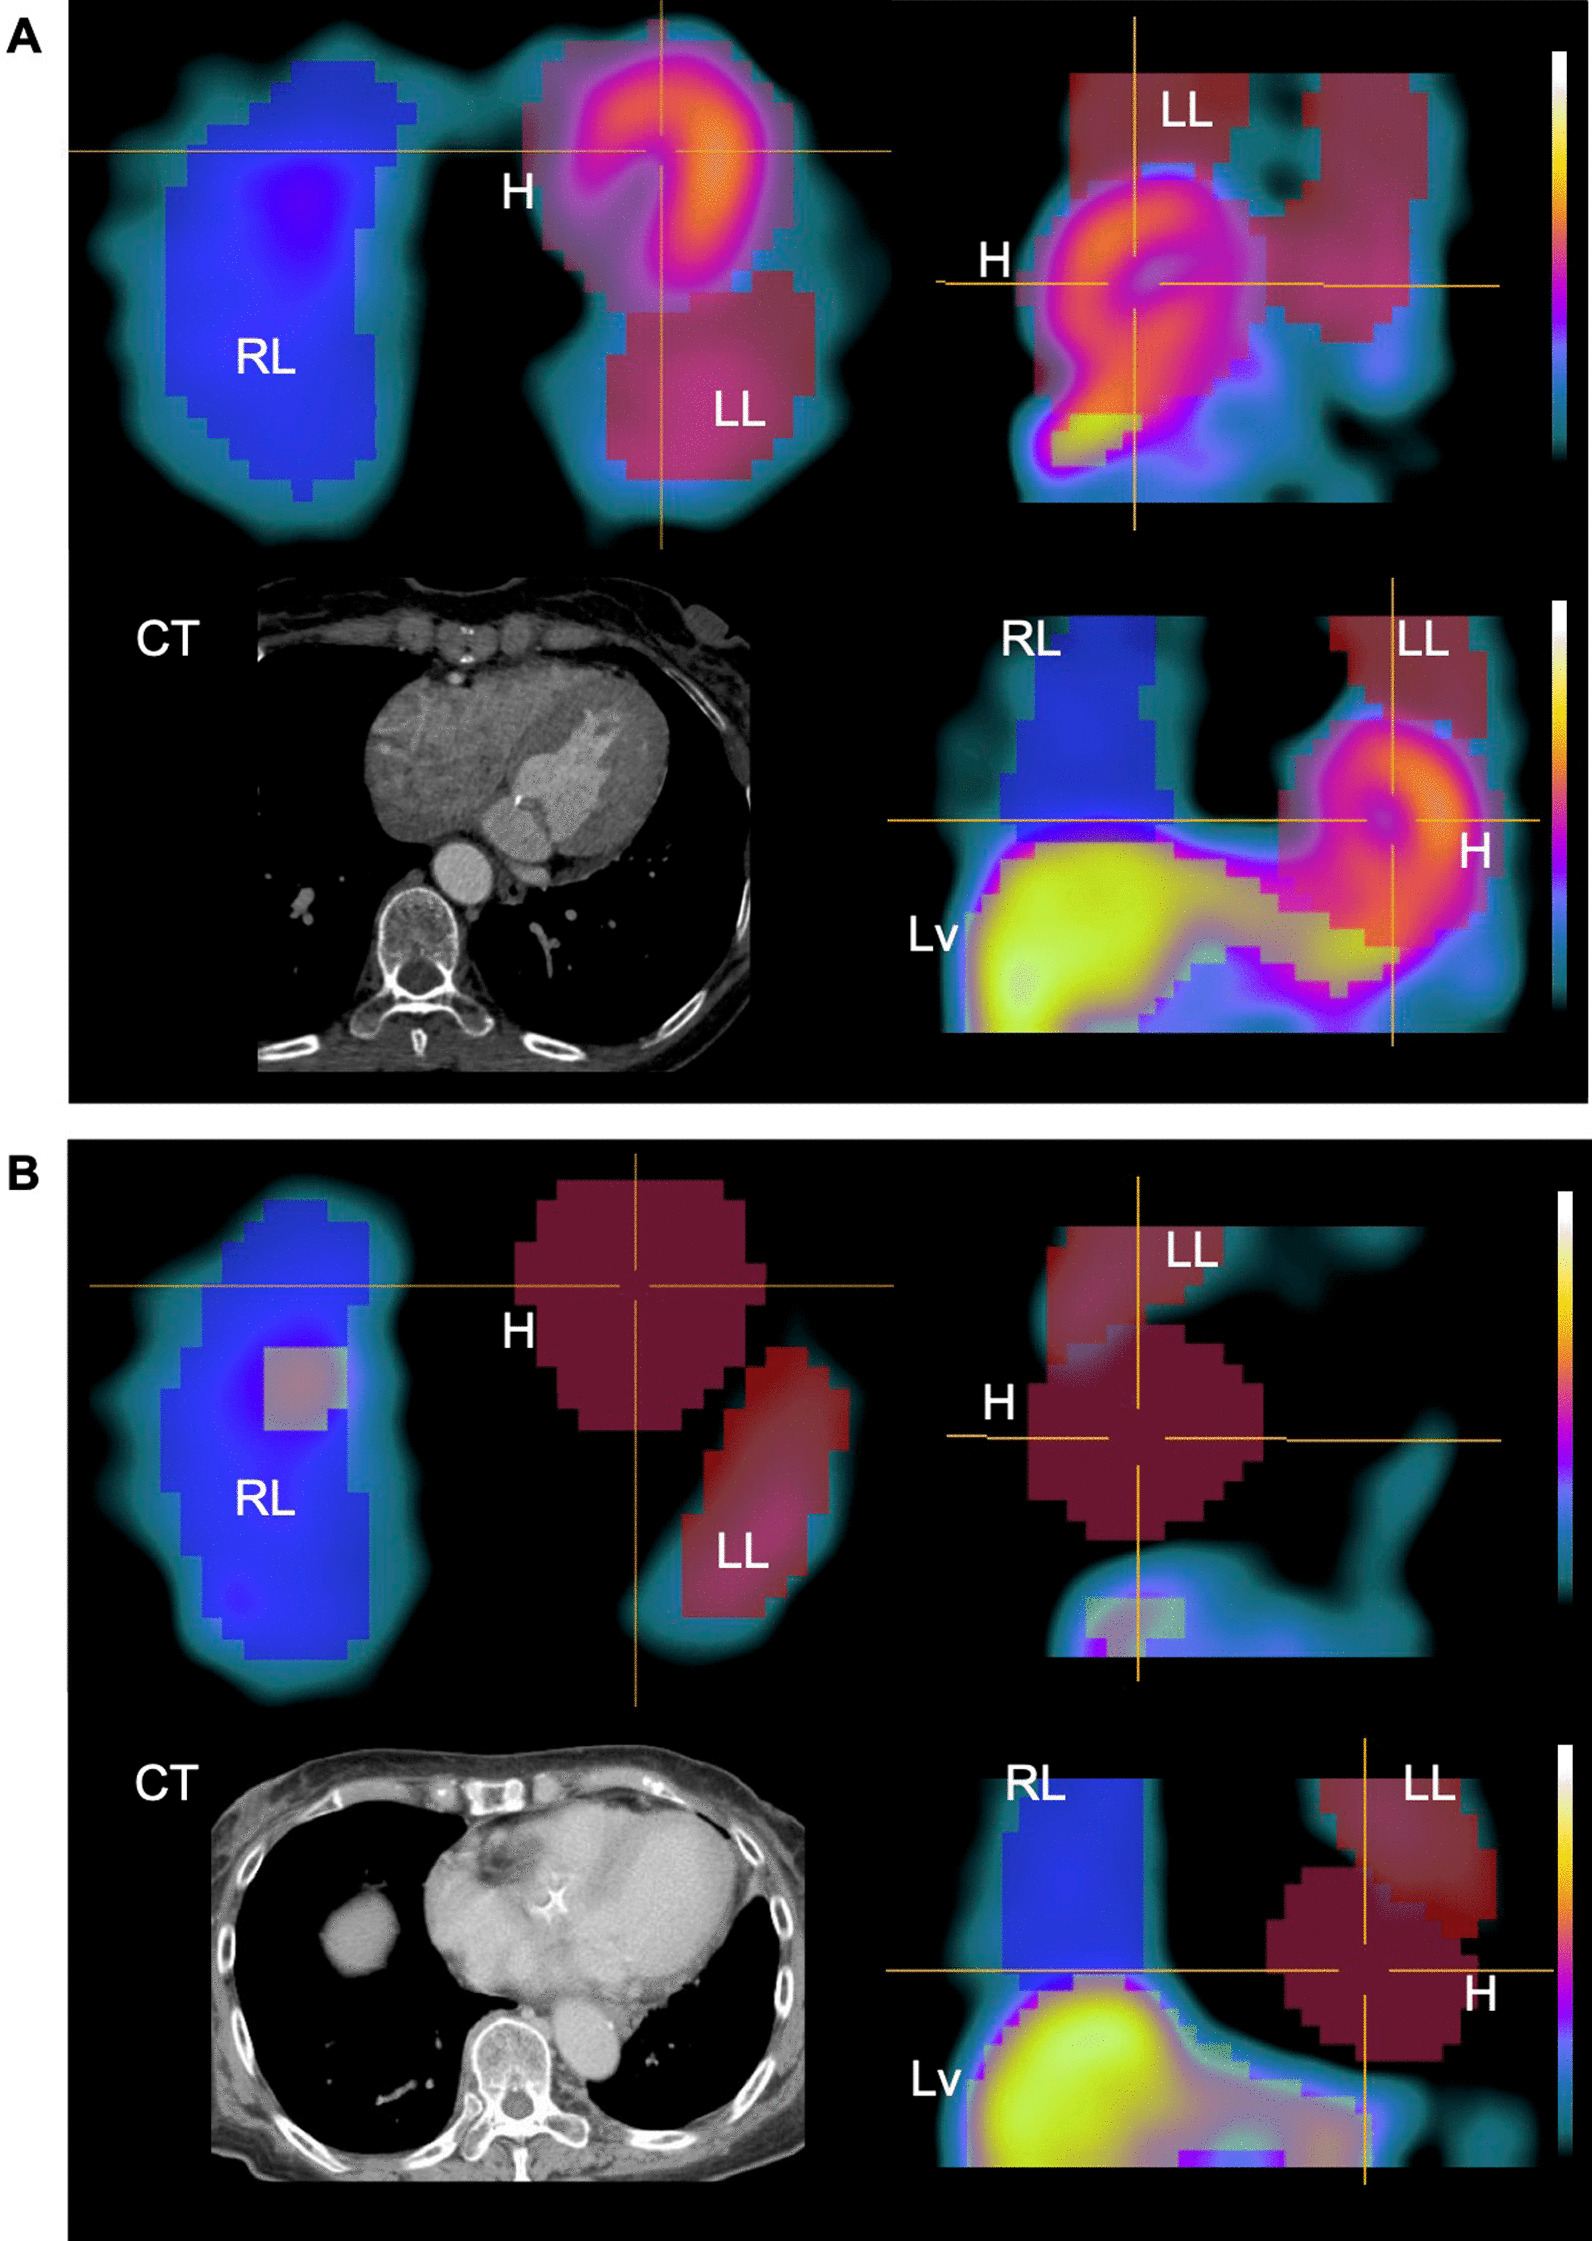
CNN-based segmentation images with ^123^I-MIBG SPECT data. Patients with normal (A) and reduced (B) uptake. Heart segmentation is correctly identified without anatomical CT images. Liver and lungs are naturally segmented as original organs. Contrast-enhanced X-ray CT images, which were performed for different purposes, are shown as an anatomical reference. H, heart; LL, left lung; Lv, liver; RL, right lung.
